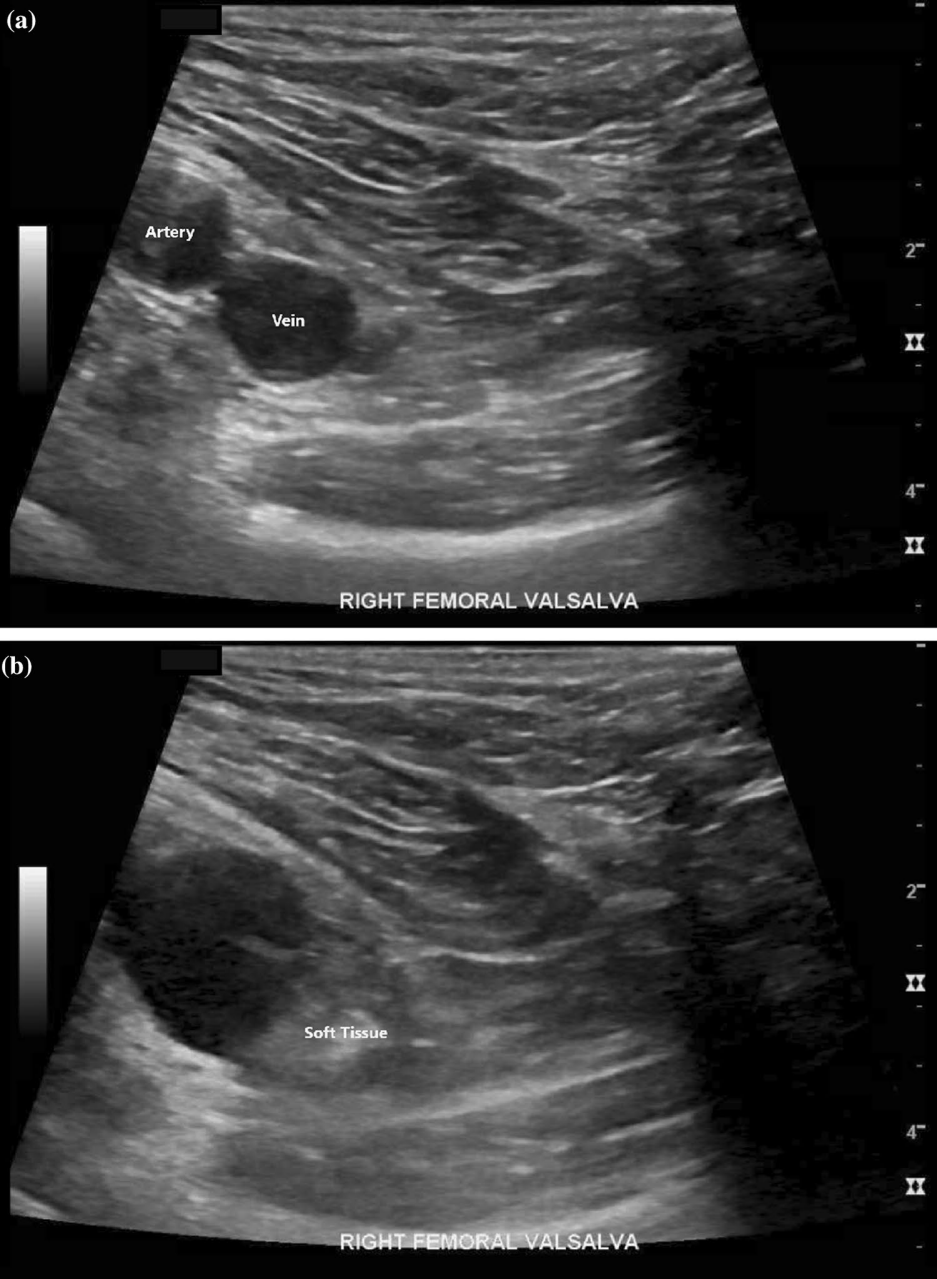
Ultrasound images demonstrating hyperechoic soft tissue protrusion medial to the femoral artery and vein within the femoral canal acquired pre-(a) and post (b)-valsalva. Femoral artery in the image is labeled as artery, and the femoral vein is labeled as vein
